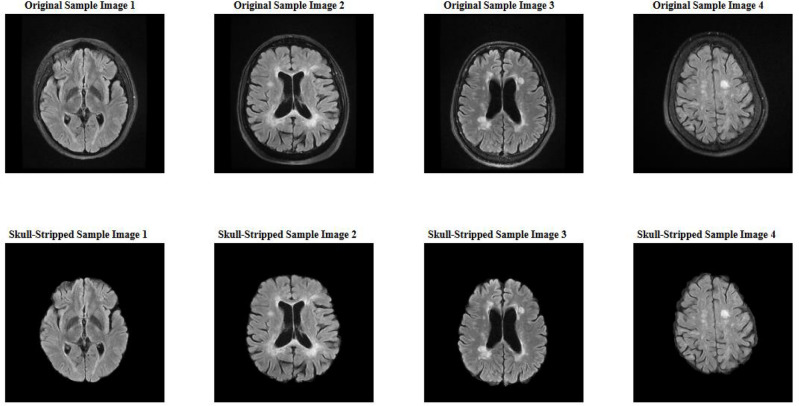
Example of a skull-stripped FLAIR MRI image.
